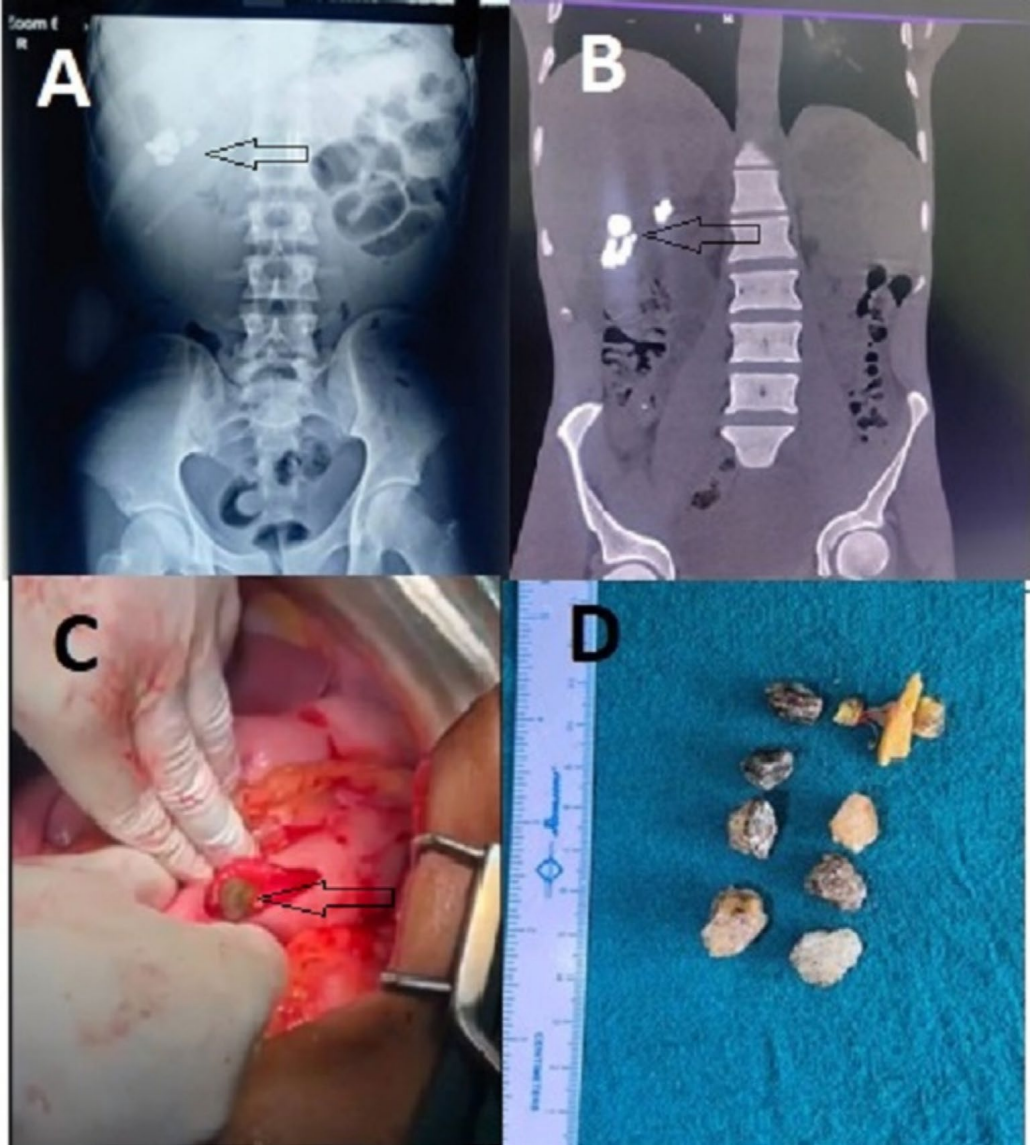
Imges of case 1; (A) X‐ray abdomen showing radiopaque findings in duodenum (Arrow); (B) CT scan of abdomen showing multiple radio opaque material in duodenum (Arrow); (C) Gastrotomy with removal of lithobezoar (Arrow); (D) Multiple lithobezoar removed from the duodenum.
